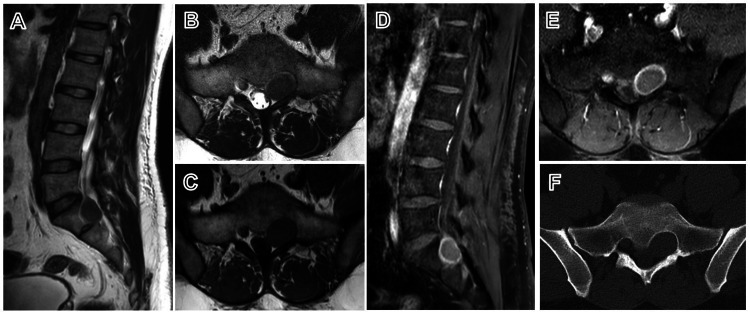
MRI of the lumbosacral spine T2 sagittal (A) and T2 axial (B) images show an isointense lesion in the left S1 neural foramen; T1 axial (C) demonstrates isointensity; T1 contrast sagittal (D) and axial (E) images show peripheral enhancement. CT axial image (F) demonstrates scalloping and widening of the left S1 neural foramen.
